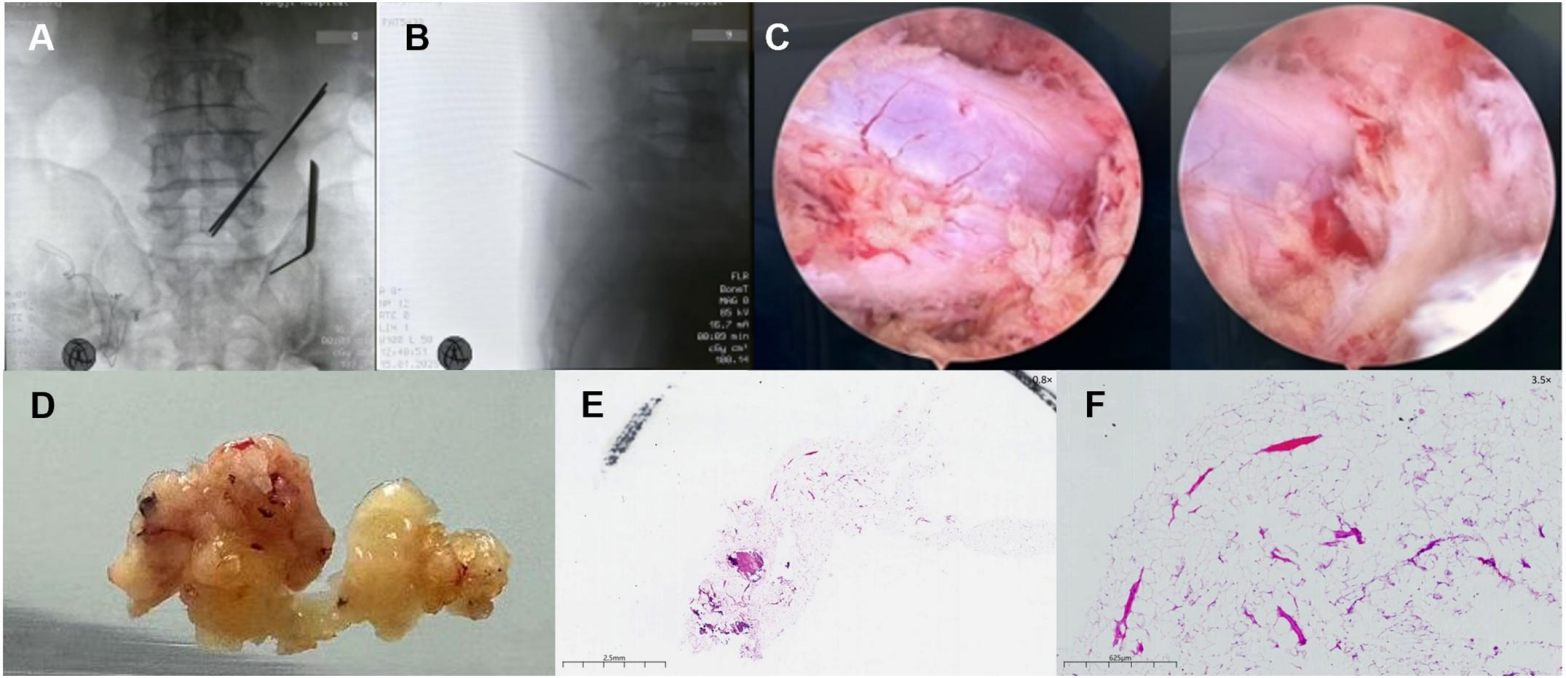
Postoperative imaging confirming effective decompression. (A,B) Postoperative CT images (sagittal and axial views) demonstrate adequate bony decompression at L5–S1. (C–E) Postoperative sagittal MRI (T1, T2, and fat-suppressed sequences) showing resolution of the epidural fat compression. Note the signal changes in the posterior soft tissue corresponding to the surgical tract. (F) Postoperative axial T2-weighted image confirms the restored patency of the spinal canal.
